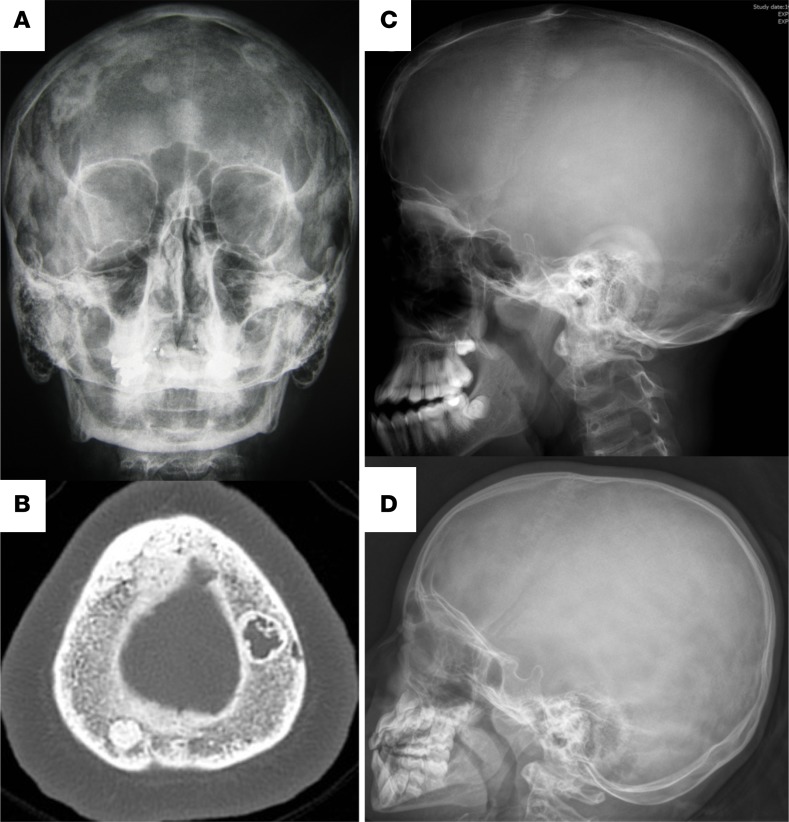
Characteristics of the skull lesions. (A) Skull radiographs of 3 patients with the p.Arg50* mutation showing extensive sclerotic lesions in patient 1-2 at adult age. (B) On computerized tomography, the lesions have mixed sclerotic and lytic appearance. Patient F2-1 has only one single sclerotic lesion at the age of 15 years (C) while patient F4-1 has normal skull radiograph at 6 years (D).
